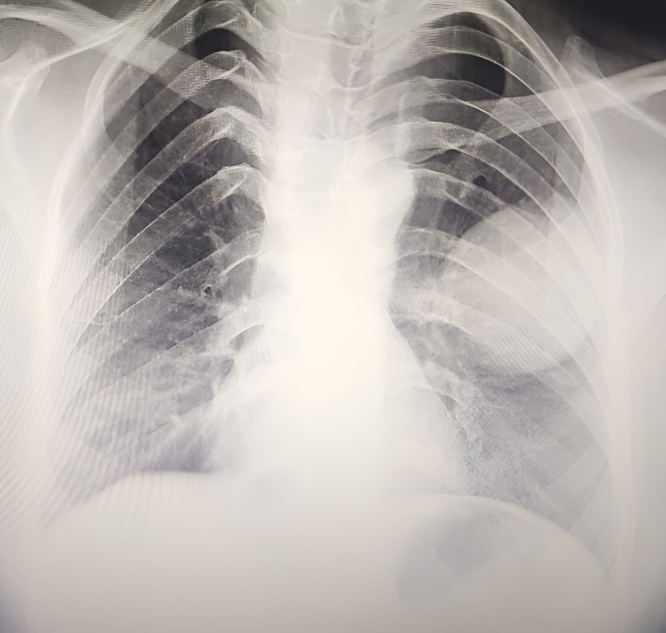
X-ray chest shows round homogenous opacity in the left middle zone of the lung.
